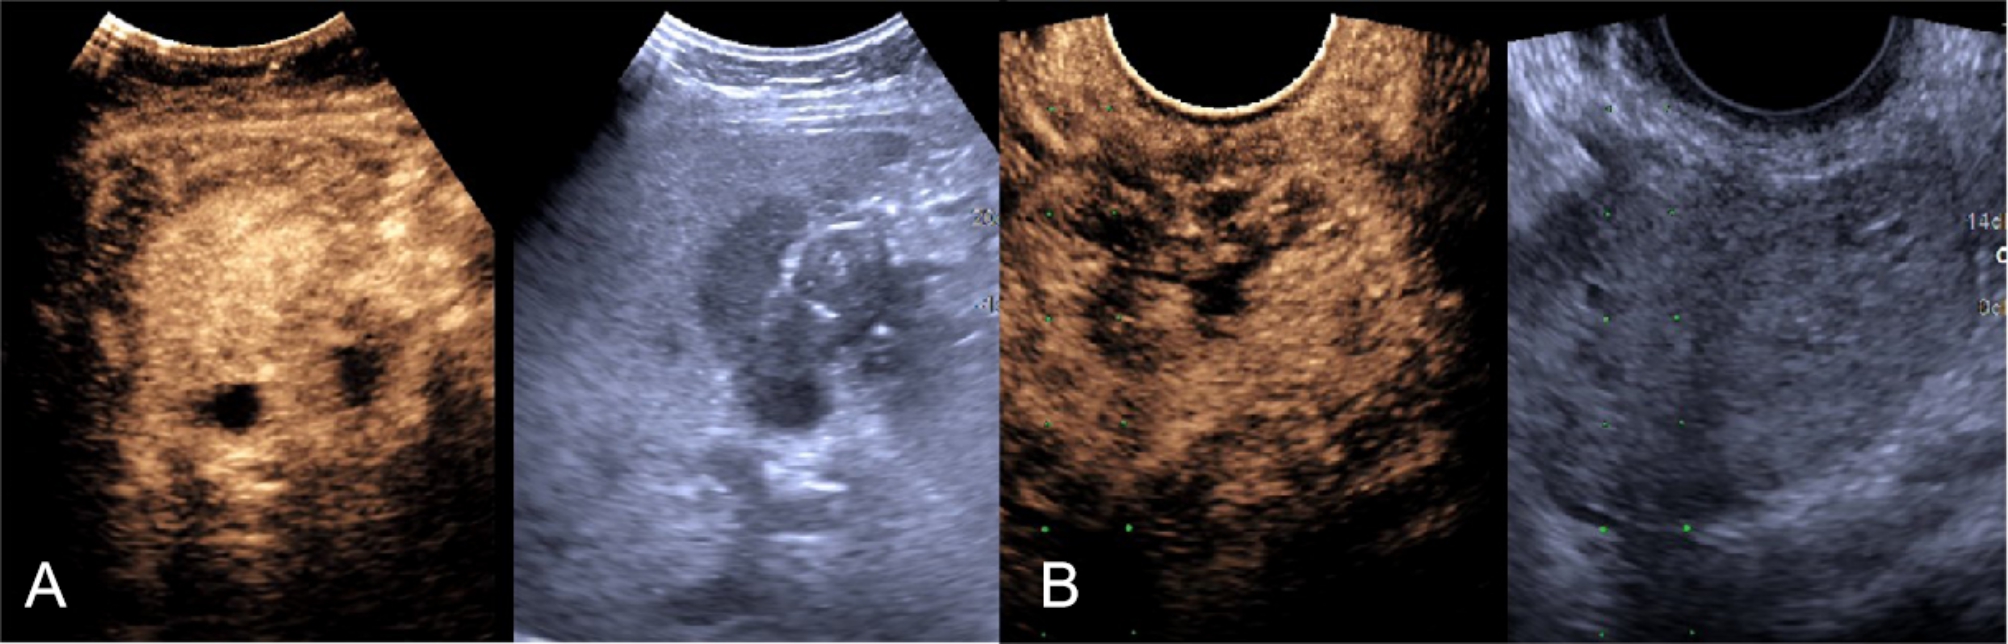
Ultrasound examination. (A) contrast-enhanced ultrasound (CEUS) showed that the gallbladder and intrahepatic hypoechoic lesion were enhanced earlier than the other parts of the liver. (B) an irregularly substantial mass was seen in the right area which showed uneven high enhancement with rapid advancement and rapid exit on CEUS
